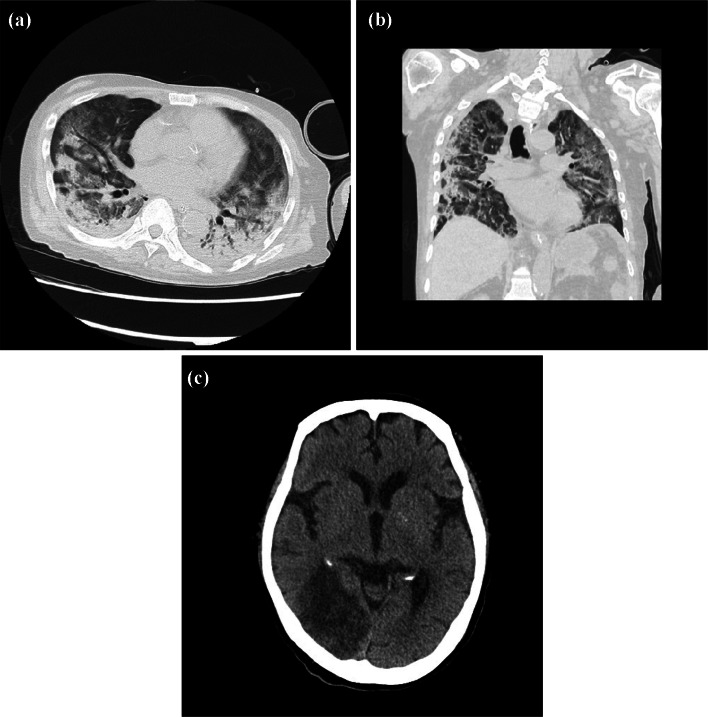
Computed tomography scans on day 26. a, b Chest computed tomography scan. c Cranial computed tomography scan
